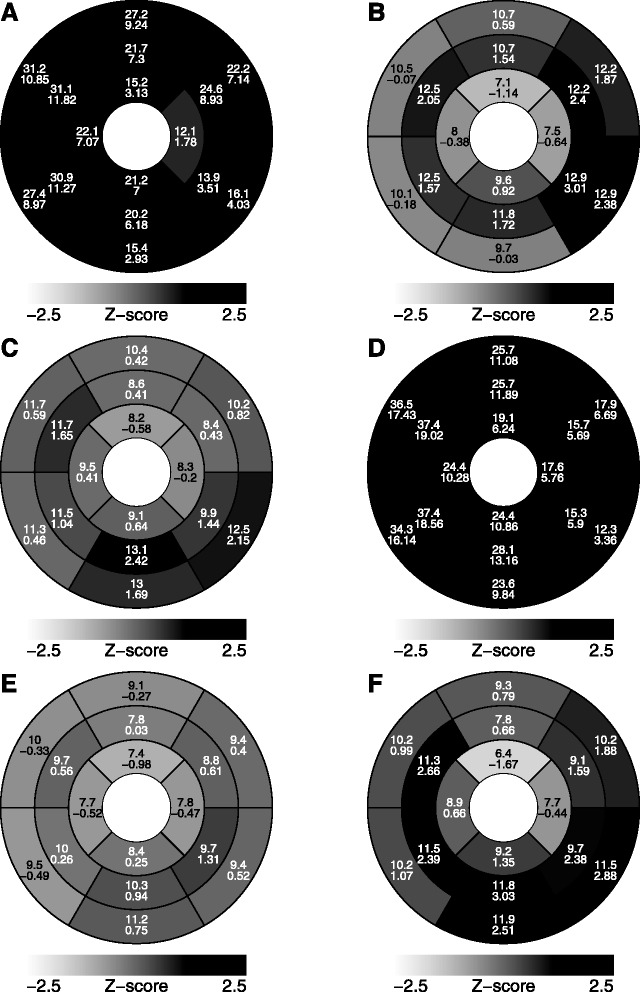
Distribution of left ventricular hypertrophy of six *PRKAG2* mutation carriers. Upper and lower values represent absolute (mm) and standardized (z-score) maximal wall thickness in the segment using adult references. Gray scaling of each segment is based on z-score. Members of family 1 with an R302Q mutation: I:1 (a), II:1 (b), II:2 (c), II:3 (d) and II:4 (e). A member of family 2 with an H344P-mutation: II:1 (f). The 16-year-old male (e), with body-surface-area (BSA) of 1.33 m2, had hypertrophy in mid-infero-lateral segment (posterior free wall) of 10 mm (z-score 2.3) using the BSA-standardized echocardiography based reference values for children
